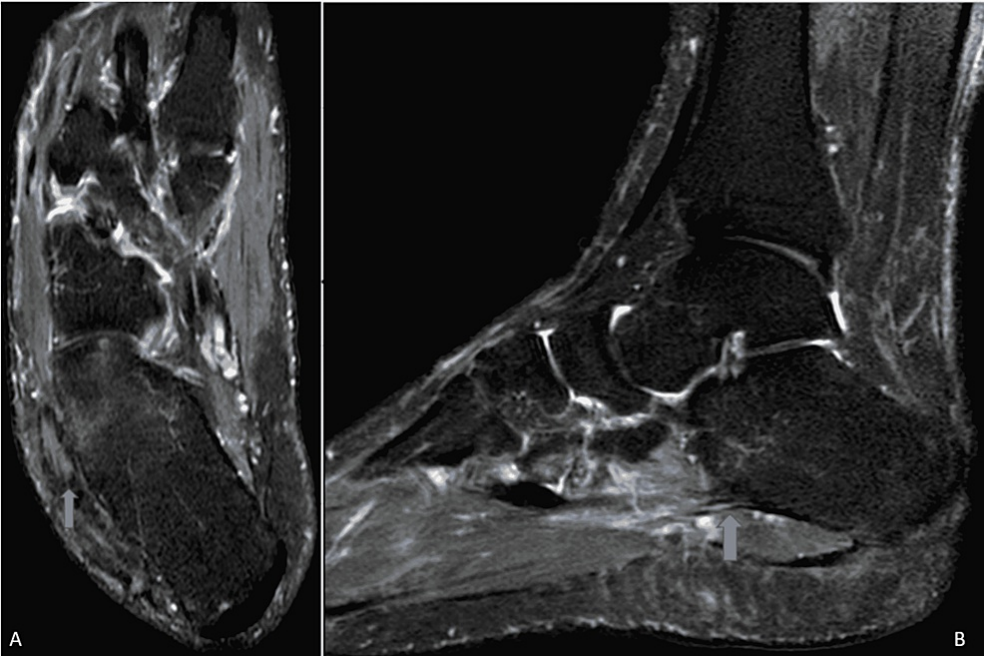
MRI images three months postoperatively revealed complete excision of the osseous lesion and a significant decrease in bone marrow edema. A: axial plane; B: sagittal plane.
